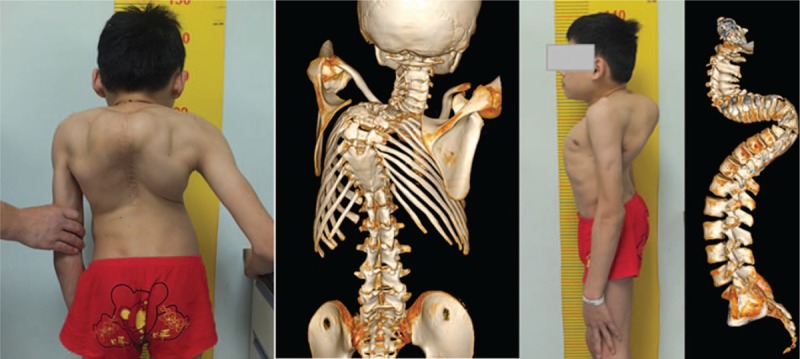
Preoperative 3D CT scans showing extremely severe postlaminectomy thoracic kyphotic deformity and posterior spinal fusion. 3D CT = three-dimensional-computed tomography.
